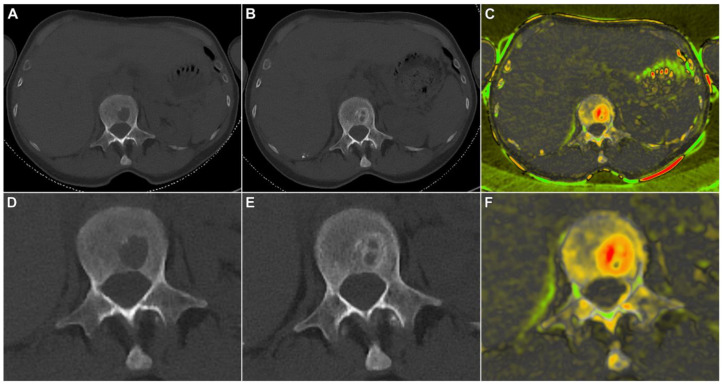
Depiction of therapy response in a 62-year-old woman with multiple myeloma (IgG kappa, R-ISS I). Baseline CT exhibited an osteolytic lesion in lumbar vertebra 1 (A,D) with trabecular sclerosis after six cycles of E-KRd (B,E). A color-coded subtraction map highlights the extent of remineralization within different parts of the lesion (C,F). Upper row: standard view. Lower row: zoomed image of the same slice.
